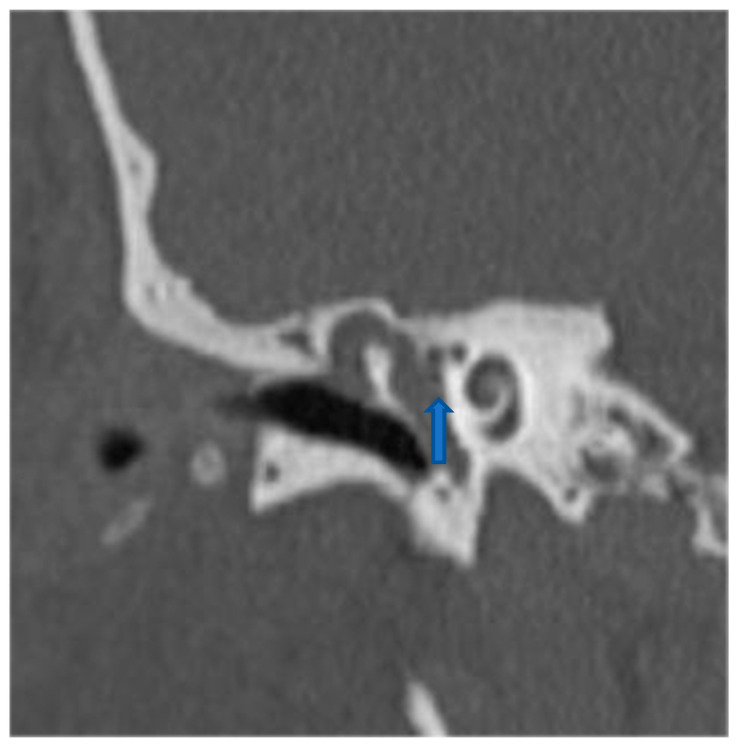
Preoperative high-resolution CT scan demonstrating cholesteatoma with partial erosion of the malleus, absence of the incus, and a dehiscence of the lateral semicircular canal consistent with lateral semicircular canal fistula (blue arrow fistulae).
